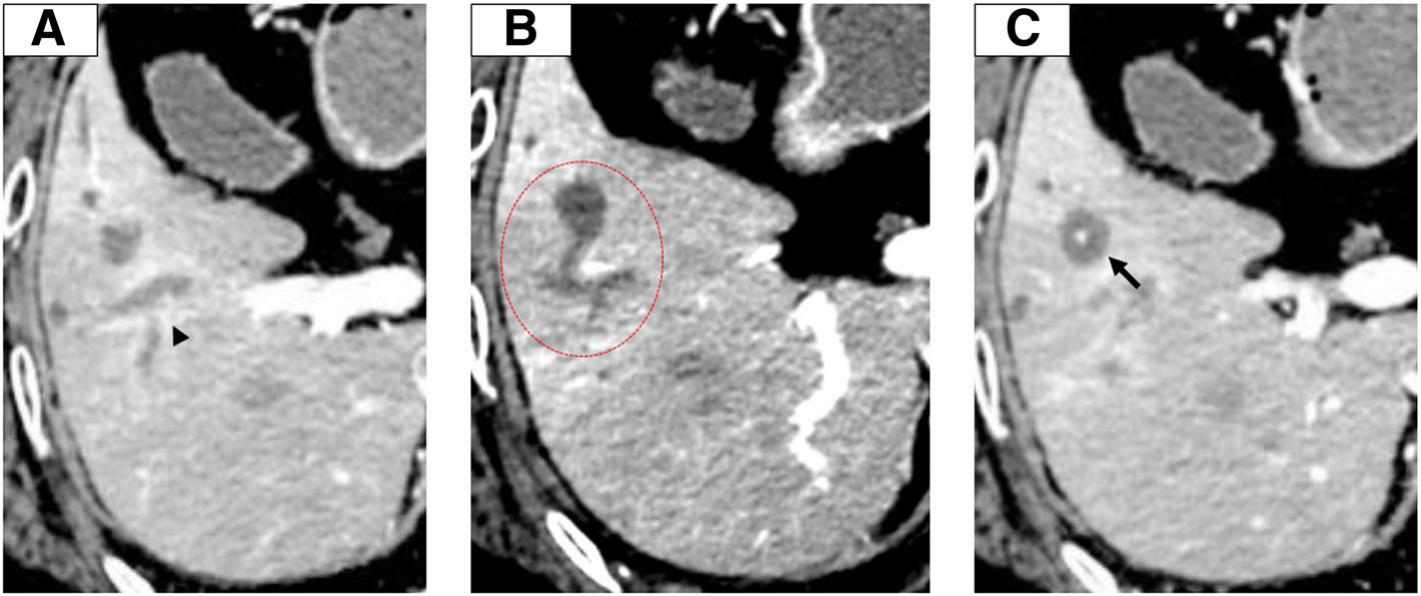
Continuous enhanced CT image from the cranial to caudal side. a: Periductal enhancement is observed along the dilated bile ducts (arrowhead). b, c: The peripheral branch of the continuous expanded bile duct exhibited a mass-like (b, red circle) region that contained a spotty high-density area that was observed even on plane-phase CT (c, arrow)
